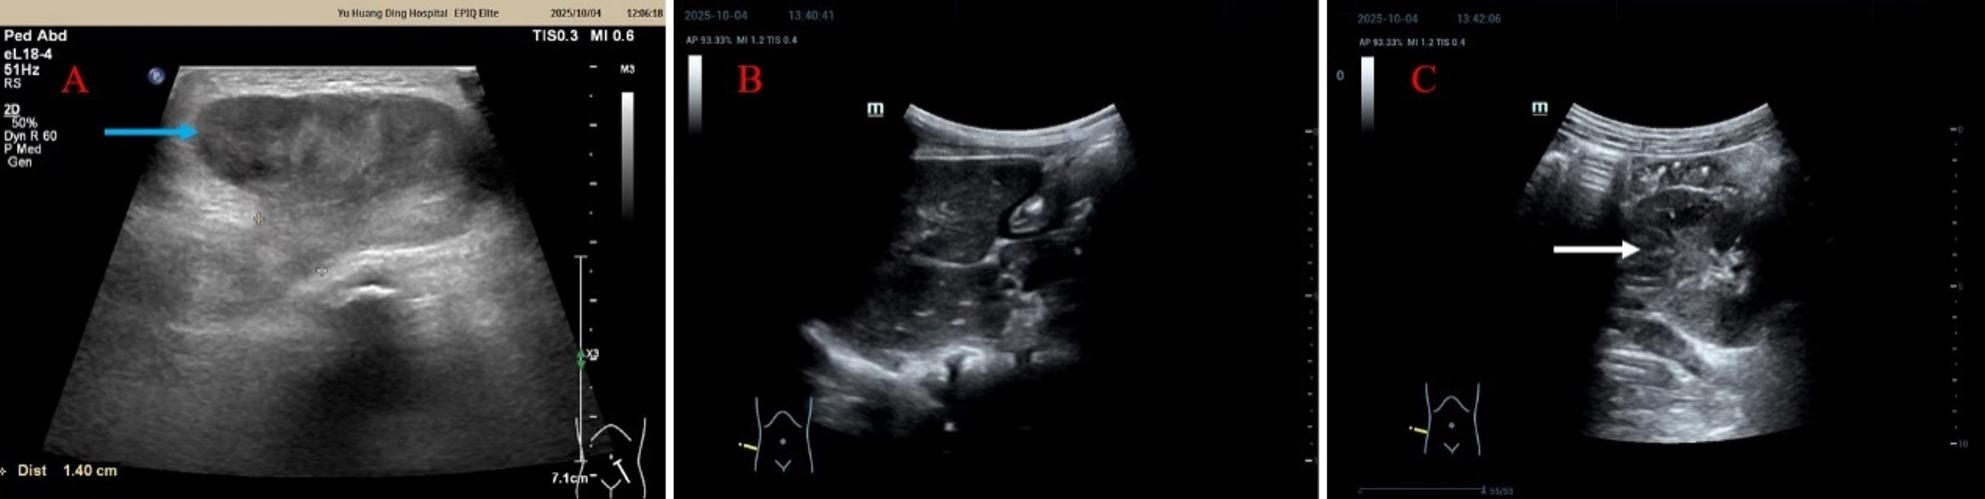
Preoperative ultrasound findings. A Longitudinal view of the left inguinal region demonstrates herniation of the ovary and fallopian tube (blue arrow), with continuity into the abdominal cavity. B Absence of renal tissue in the right renal fossa on urinary system ultrasound. C Transverse image of the right lower quadrant reveals an ectopic kidney with preserved renal capsule and intact collecting system (white arrow)
